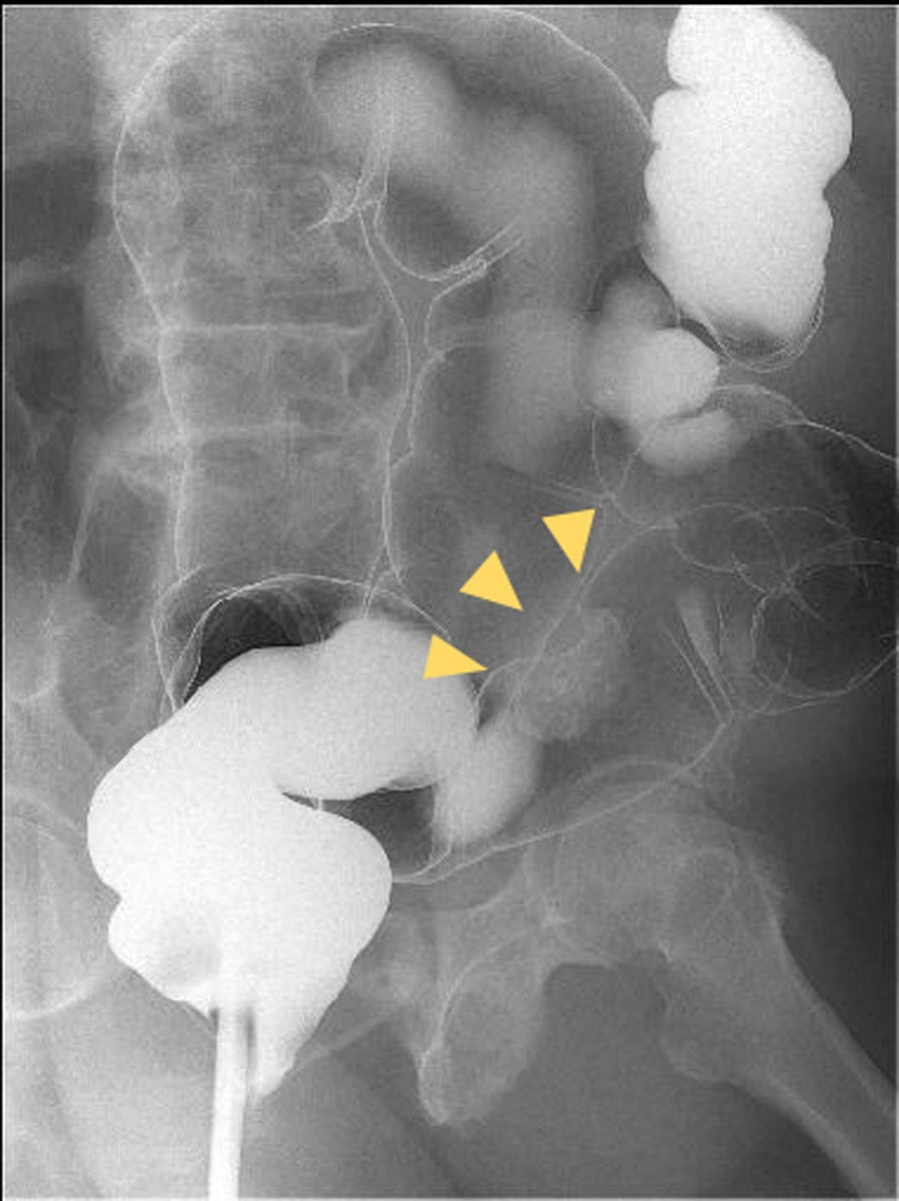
Radiographic contrast enema findings. A 3-cm stricture can be seen in the sigmoid colon, and the apple core sign is positive (yellow arrow)
